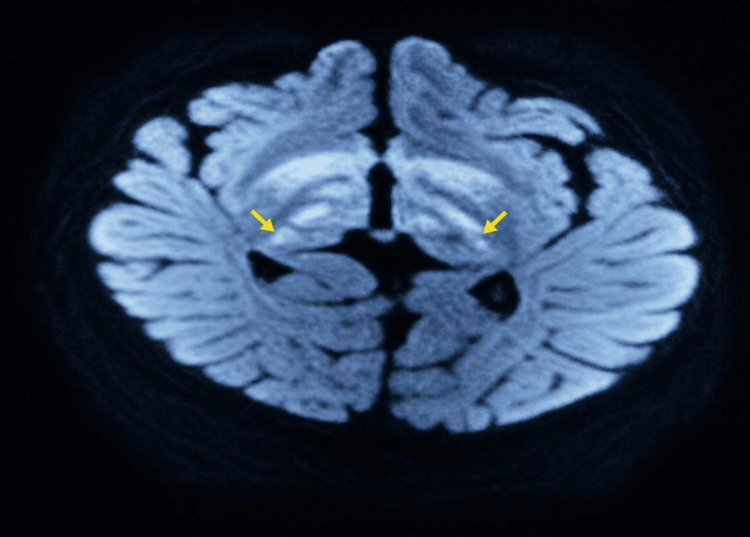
Axial FLAIR brain magnetic resonance imaging The Fluid-Attenuated Inversion Recovery (FLAIR) MRI image demonstrates bilateral symmetrical hyperintensity of the basal ganglia, predominantly involving the thalami (yellow arrows). These neuroradiological findings initially raised suspicion of mitochondrial encephalopathy, particularly Leigh syndrome.
